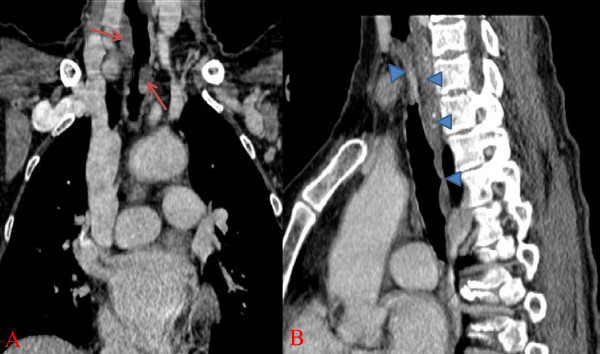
Tracheal computed tomography. A circumferential tracheal tumor caused a reduction of her caliber.
